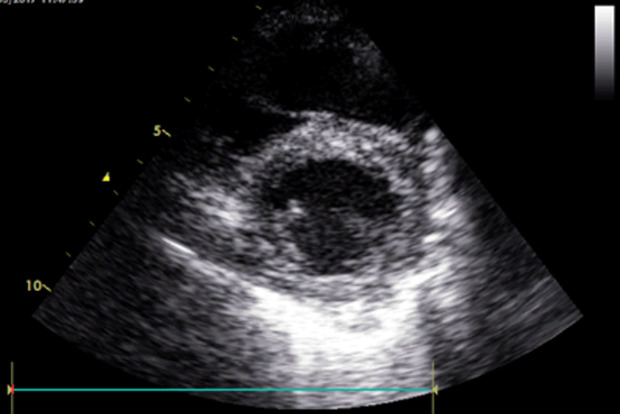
follow-up transthoracic echocardiogram after adenotonsillectomy, revealing diminished right ventricle dimensions
